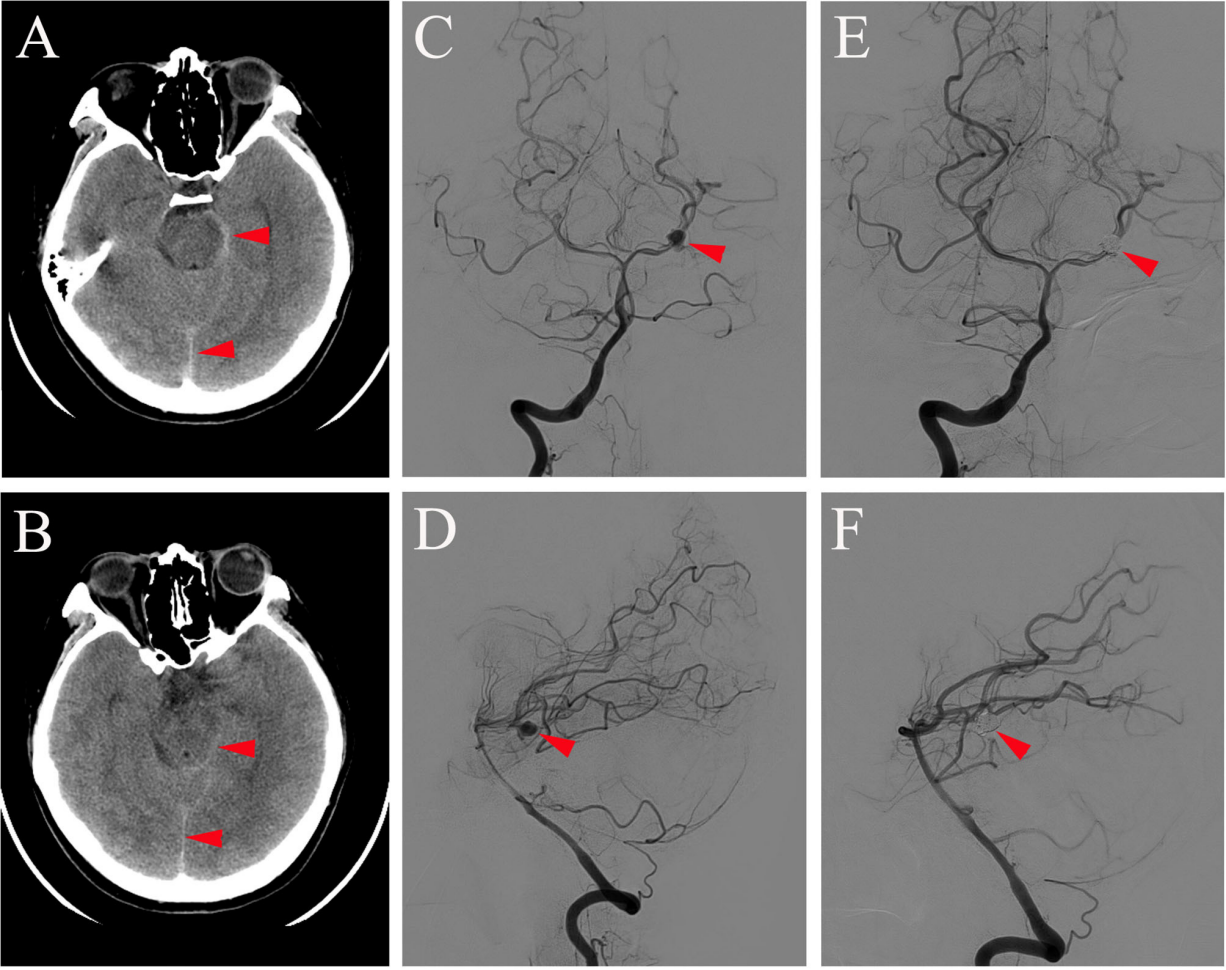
Brain CT (A,B) conducted on the day of symptom onset showing subarachnoid hemorrhage (red arrowhead on A,B) and DSA of antero-posterior view (C,E) and lateral view (D,F) obtained 7 days after symptom onset showing aneurysm at the P1-P2 segment of the left PCA before (red arrowhead on C,D) and after coil embolization (red arrowhead on E,F).
